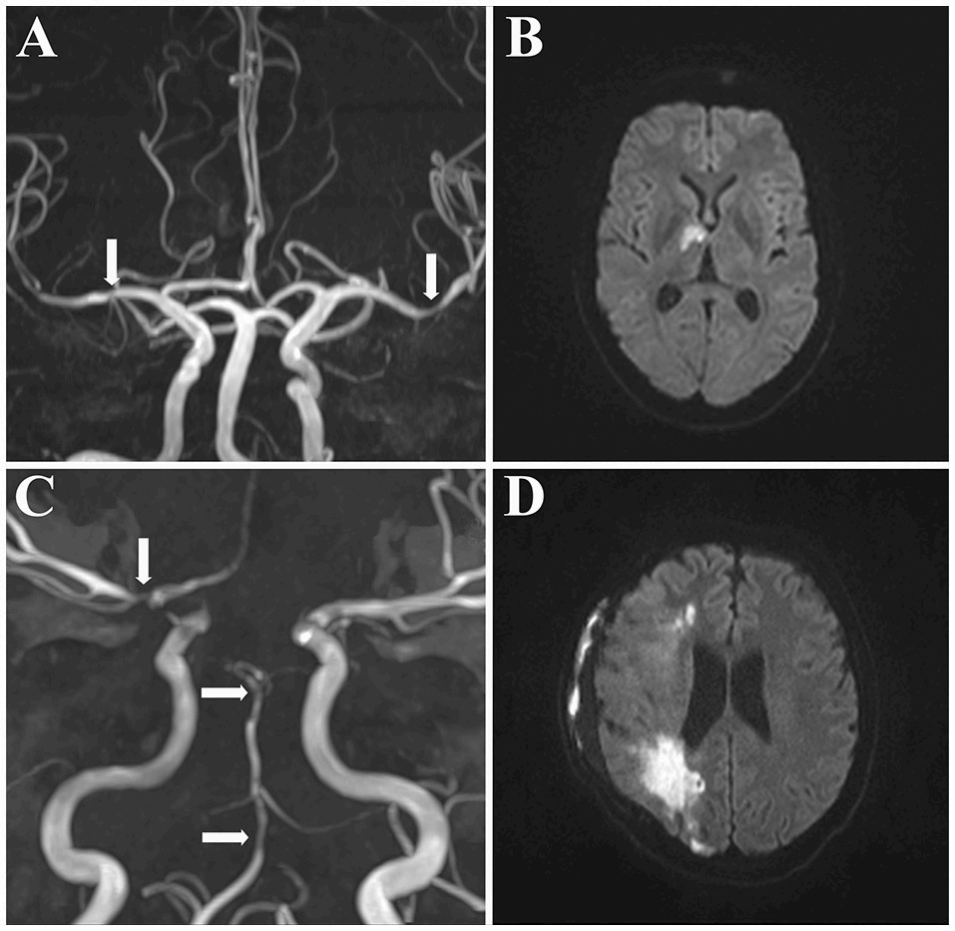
MR angiography of 2 patients with cerebral vasculitis. MR angiography with multiple vessel stenoses suggesting cerebral vasculitis. Both patients had CSF-pleocytosis (cell count 73 Mpt/L and 16 Mpt/L, respectively) and were diagnosed to have neuroborreliosis (A,B) or neurosyphilis (C,D). Arrows indicate caliber irregularities of the right middle cerebral artery (A) or the basilar artery (C) on TOF-MRA. (B,D) indicate corresponding DWI-lesions. TOF-MRA indicates Time-of-Flight magnetic resonance angiography; DWI, diffusion weighted imaging; MR, magnetic resonance.
